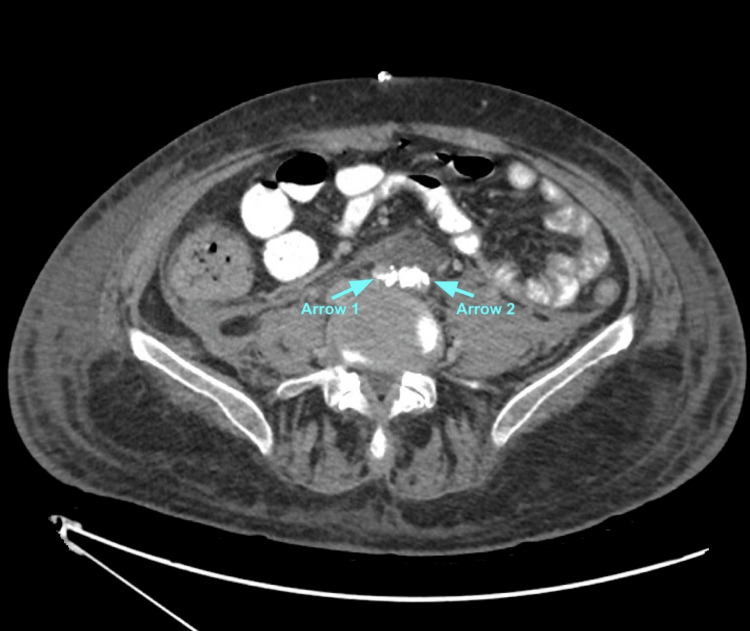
Pooling of Blood Due to Stenosis and Occlusion on CT Urogram Arrow 1: right CIA; Arrow 2: left CIA
